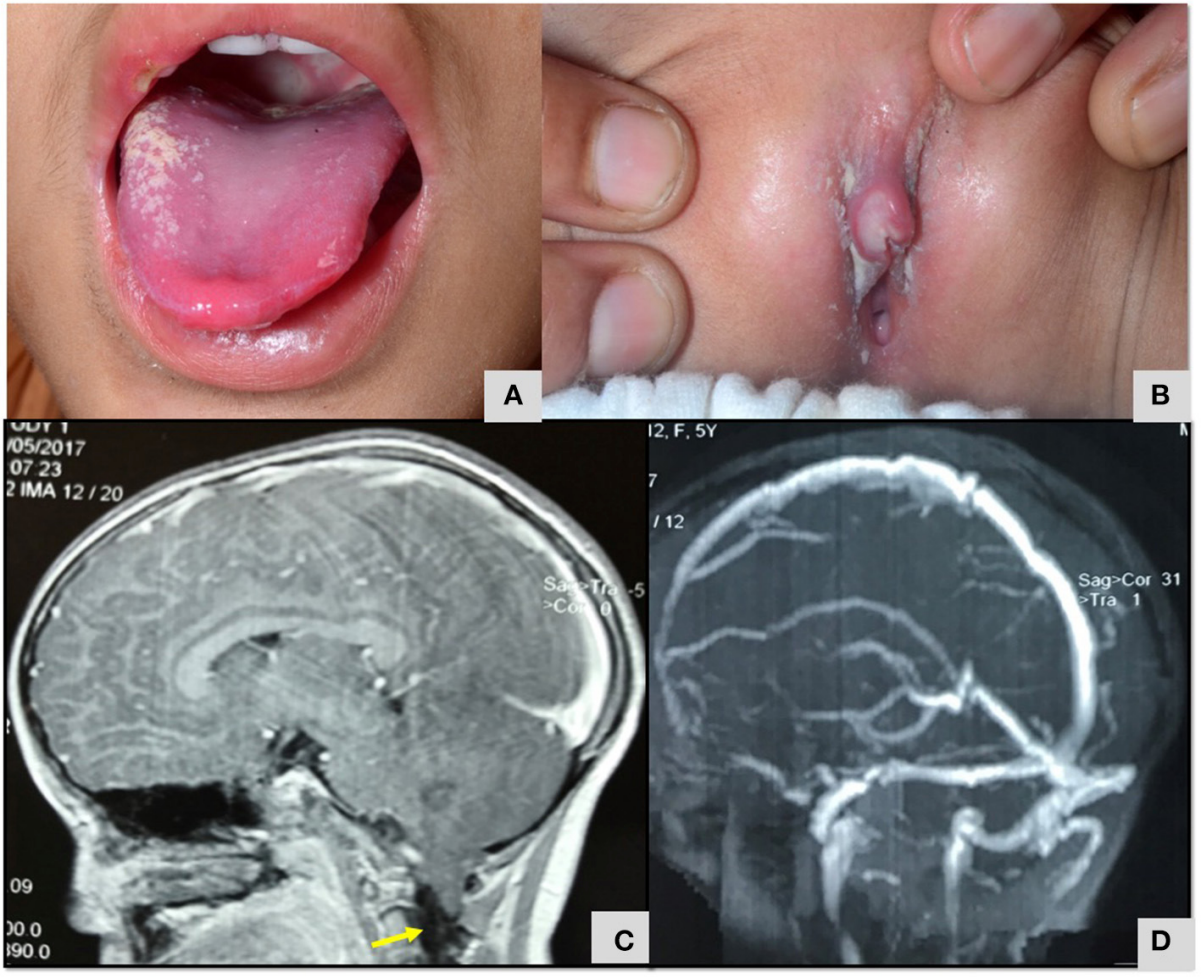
(A,B) Oral cavity erythema and ulceration and genital ulcers in child with A20 haploinsufficiency (Table 3; Patient no 40). (C) MRI Brain T2 weighted sagittal section images demonstrating type 2 Arnold Chiari malformation (Table 3; Patient no 40). (D) MR venography of brain demonstrated normal flow and no evidence of cerebral sinus venous thrombosis (Table 3; Patient no 40).
